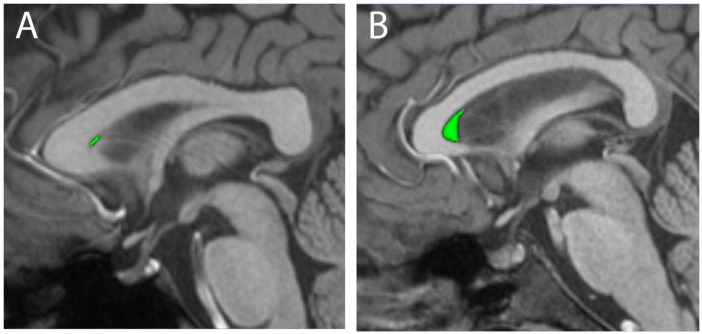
Three-dimensional renderings of manually segmented cava septa pellucida (CSP, green) are shown for representative small (A) and large (B) examples, overlaid on mid-sagittal T1-weighted MRI scans.
